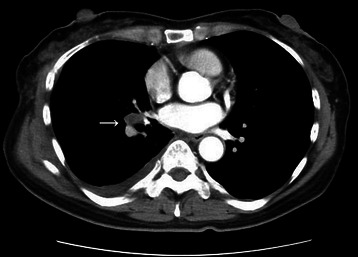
Postoperative CT scan showing the aneurysm decrease in size and thrombotic change
